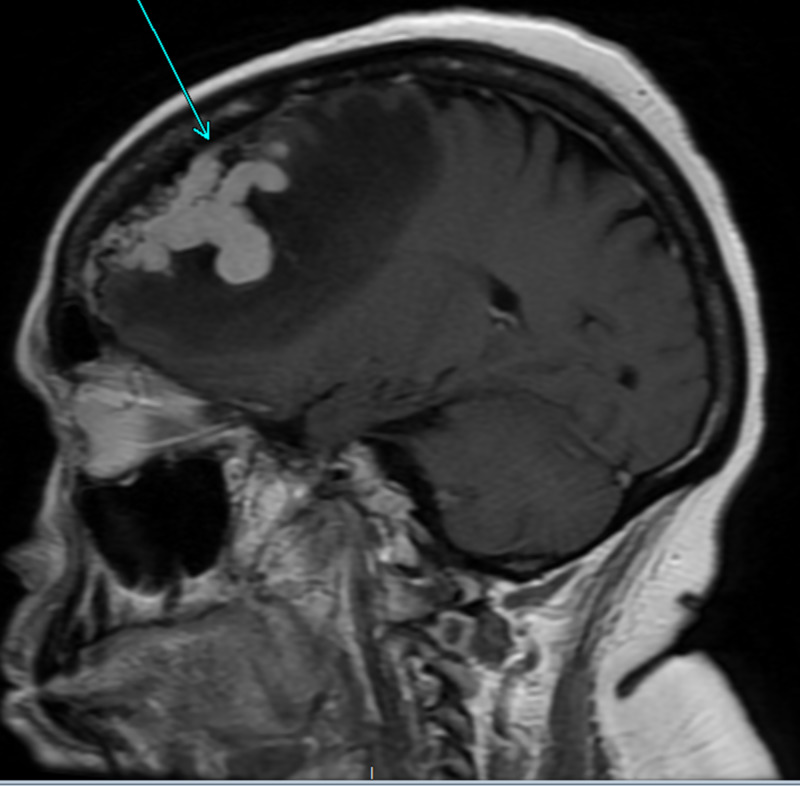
MRI brain with contrast sagittal view T1 sequence of left frontal lobe growth with surrounding vasogenic edema.
